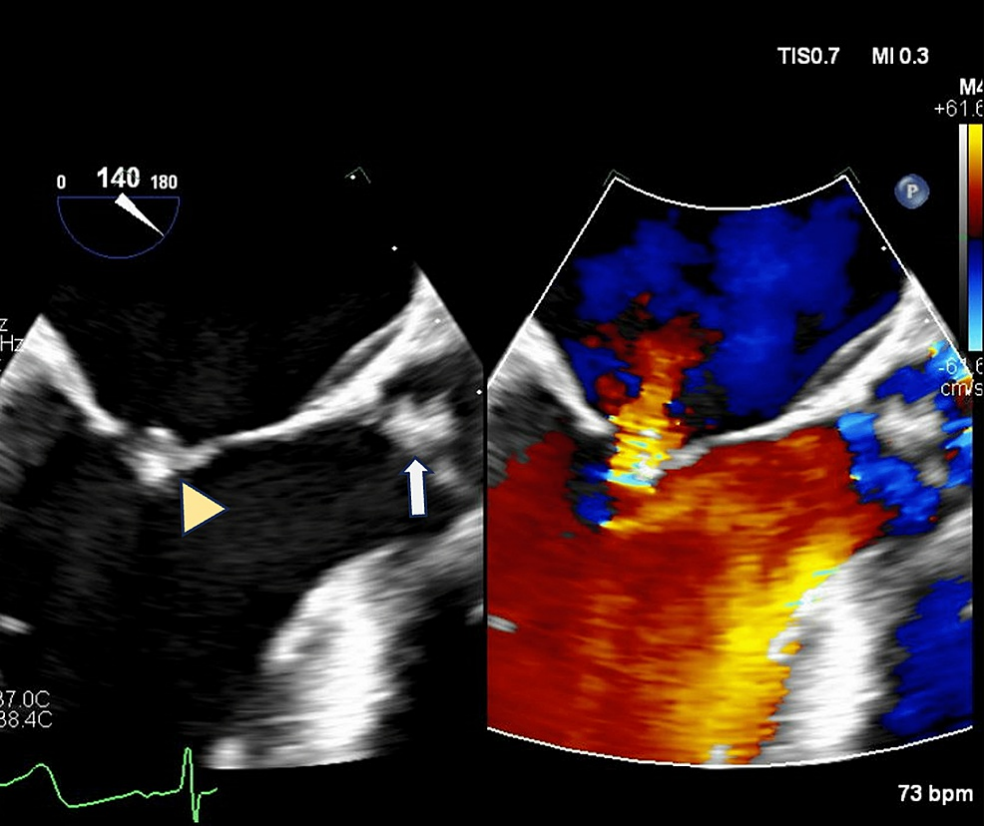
Transesophageal echocardiogram view of vegetation in mitral (arrowhead) and aortic (white arrow) valves. Doppler-colored blood flow through mitral valve is also shown in the figure.
